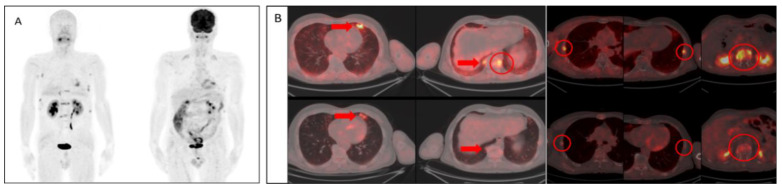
Patient No 1. 58 year-old-male diagnosed with MM. (A) Visual comparison of maximum intensity projections of both modalities (Left [68Ga]FAPI, right [18F]FDG) showed superior image quality and higher specific activity retentions with [68Ga]FAPI PET/CT. (B) In axial plane evaluations, both imaging methods demonstrated extramedullary involvement of MM in the lungs (Arrows); however, SUV_max_ values of the lesions were noted to be higher in [68Ga]FAPI PET/CT (Upper row). Also, significantly higher activity uptake was observed in the bone lesions (Circles) with [68Ga]FAPI (Upper row) when compared to [18F]FDG (Lower row).
